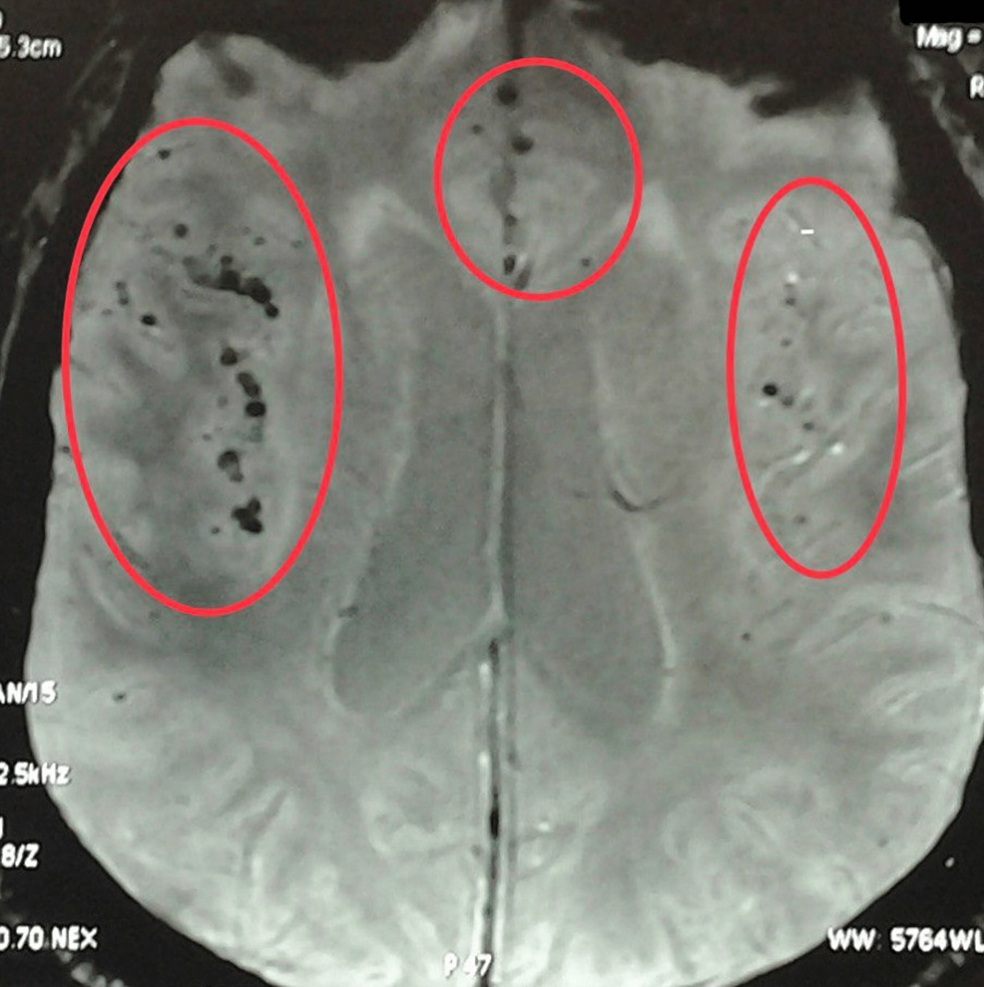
Susceptibility weighted magnetic resonance imaging of the brain showing microhemorrhages in bilateral frontoparietal regions (red circles).
